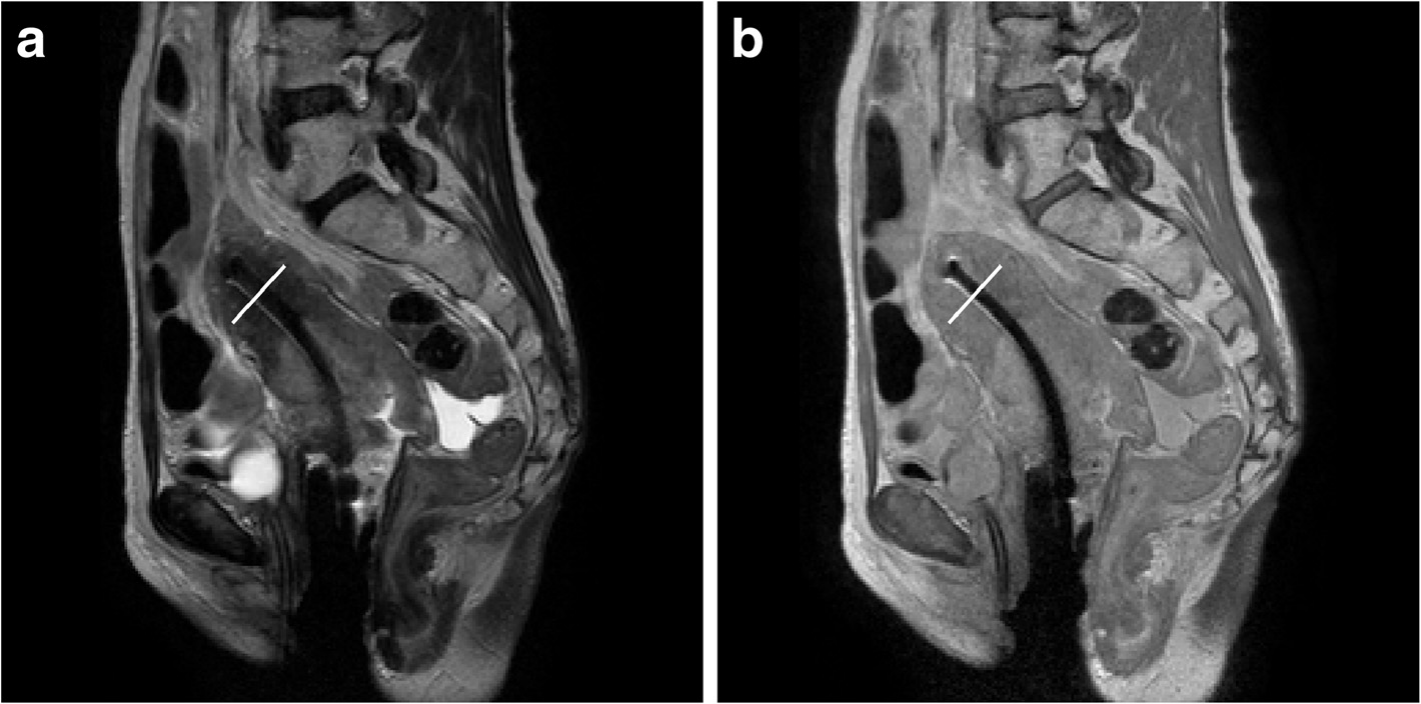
Comparison of (a) T2W and (b) PDW MRI images for localizing tumors and the applicator. White lines across the tandem on both images were added during the post processing to show the location of signal profiles plotted in Figure 2.
